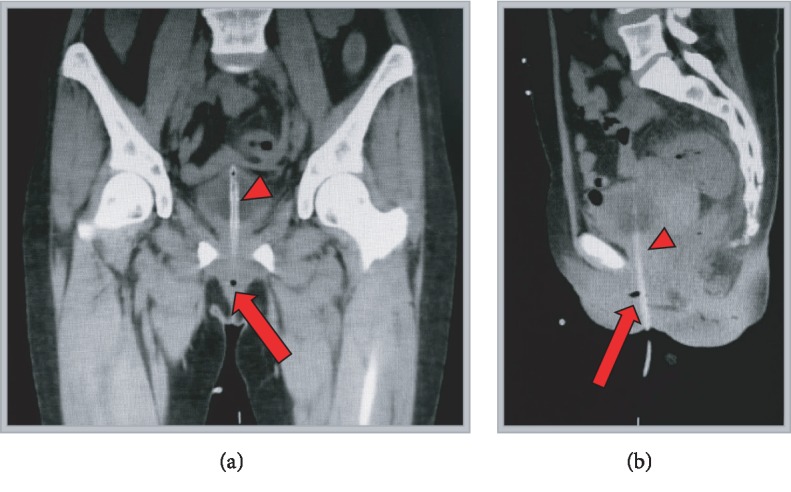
(a, b) Coronal and sagittal CT sections demonstrating the urethral defect (*red arrow*) near the in place Foley catheter (*red arrowhead*).
